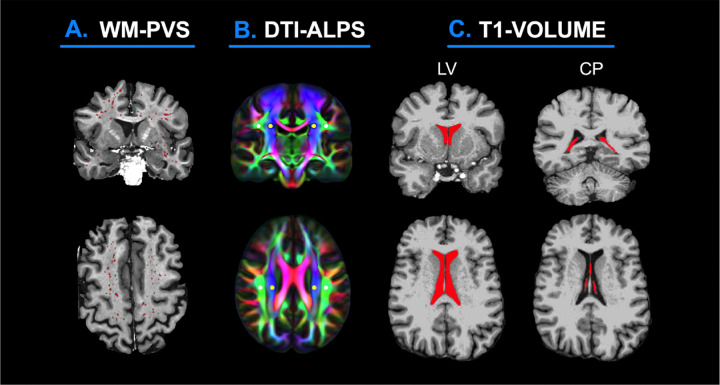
Coronal and axial MRI depictions of multimodal methods used in this study to examine CSF-based mechanisms. (A)Automated segmentation of white matter perivascular spaces (WM-PVS). (B)Diffusion tensor imaging analysis along the perivascular space (DTI-ALPS). (C)Volumetric segmentation of the lateral ventricles (LV) and choroid plexus (CP).
